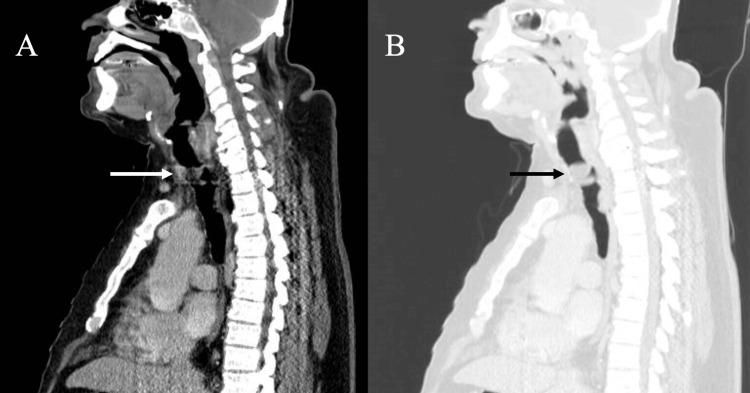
(A, B) Sagittal view of the CT revealing tracheal tumor in the abdomen and lung window, respectively (white and black arrows).
